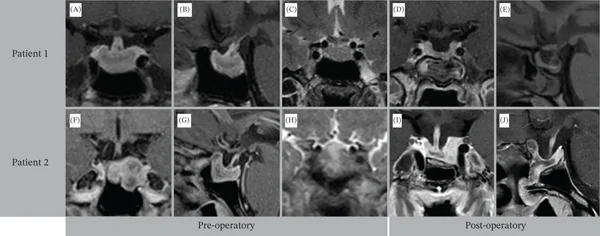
Preoperative and postoperative MRI scans of (A–E) Patient 1 and (F–J) Patient 2. Patient 1: Preoperative MRI (T1‐weighted, contrast‐enhanced: (A) coronal; (B) sagittal) shows a 16 × 11 *m*
